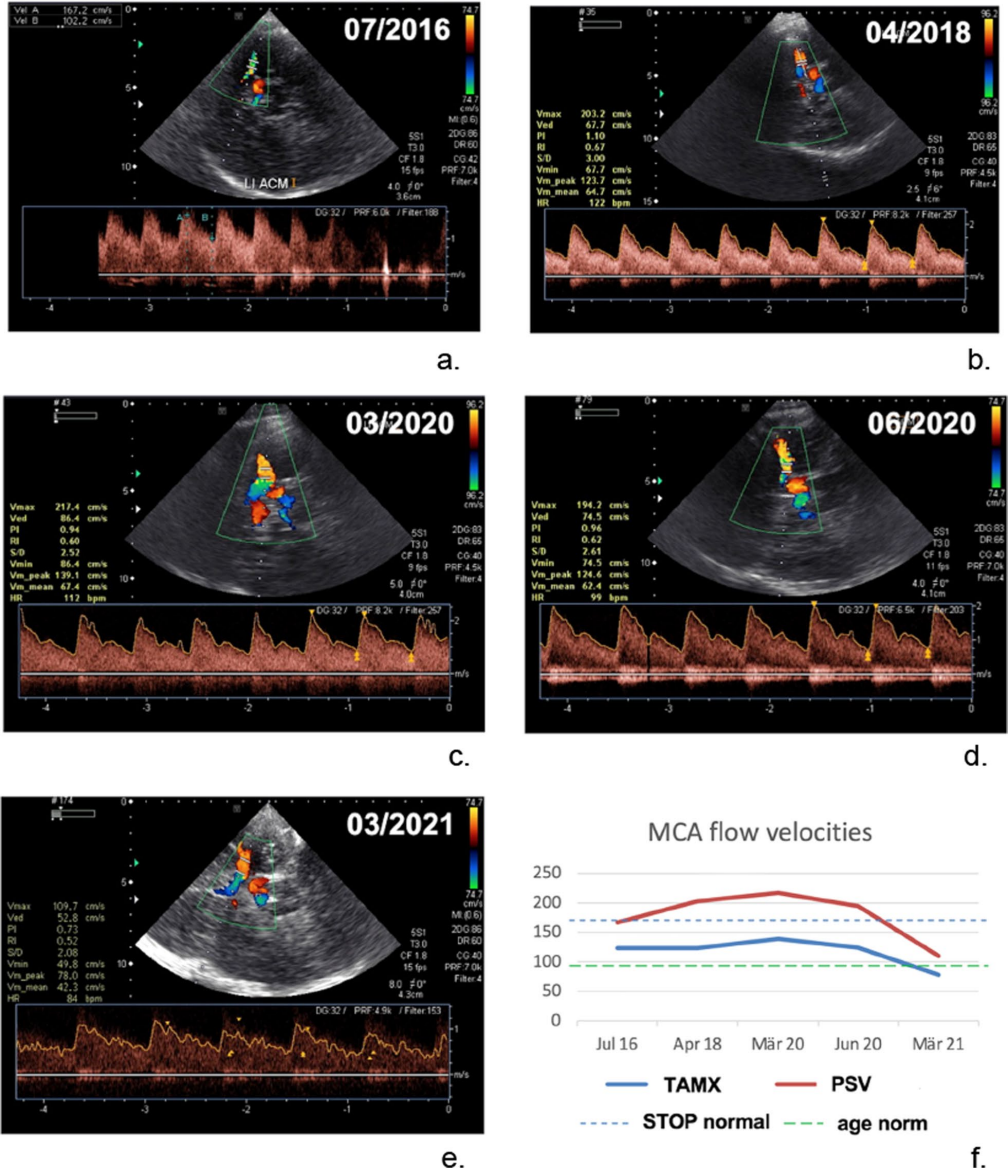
Transcranial Doppler sonography of a 14-year-old SCD-patient without stem cell transplantation. Measurements were taken in the middle cerebral artery (MCA). There was a long-term follow-up from 07/2016 to 03/2021 (see a–e). A total of 5 TCDs were performed. f Shows the time course of PSV (peak systolic velocity) and TAMX (time averaged maximum velocity, TAMMV). As abnormal blood flow velocities with a TAMX of 217 cm/s were derived in the TCD in 03/2020, we performed a control in short-term interval in 06/2020. Here, a TAMX/TAMMV of < 200 cm/s ("conditional") was shown again
